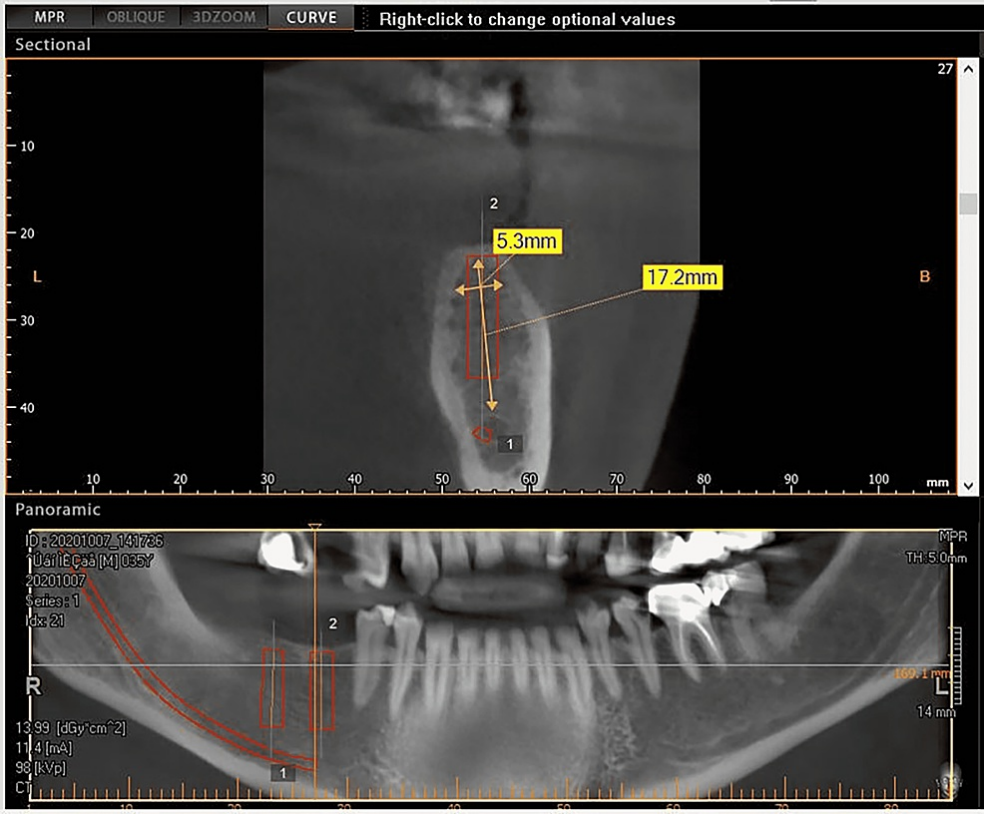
Planning implant position and determining the diameter and length of the implant using cone-beam computed tomography imaging
